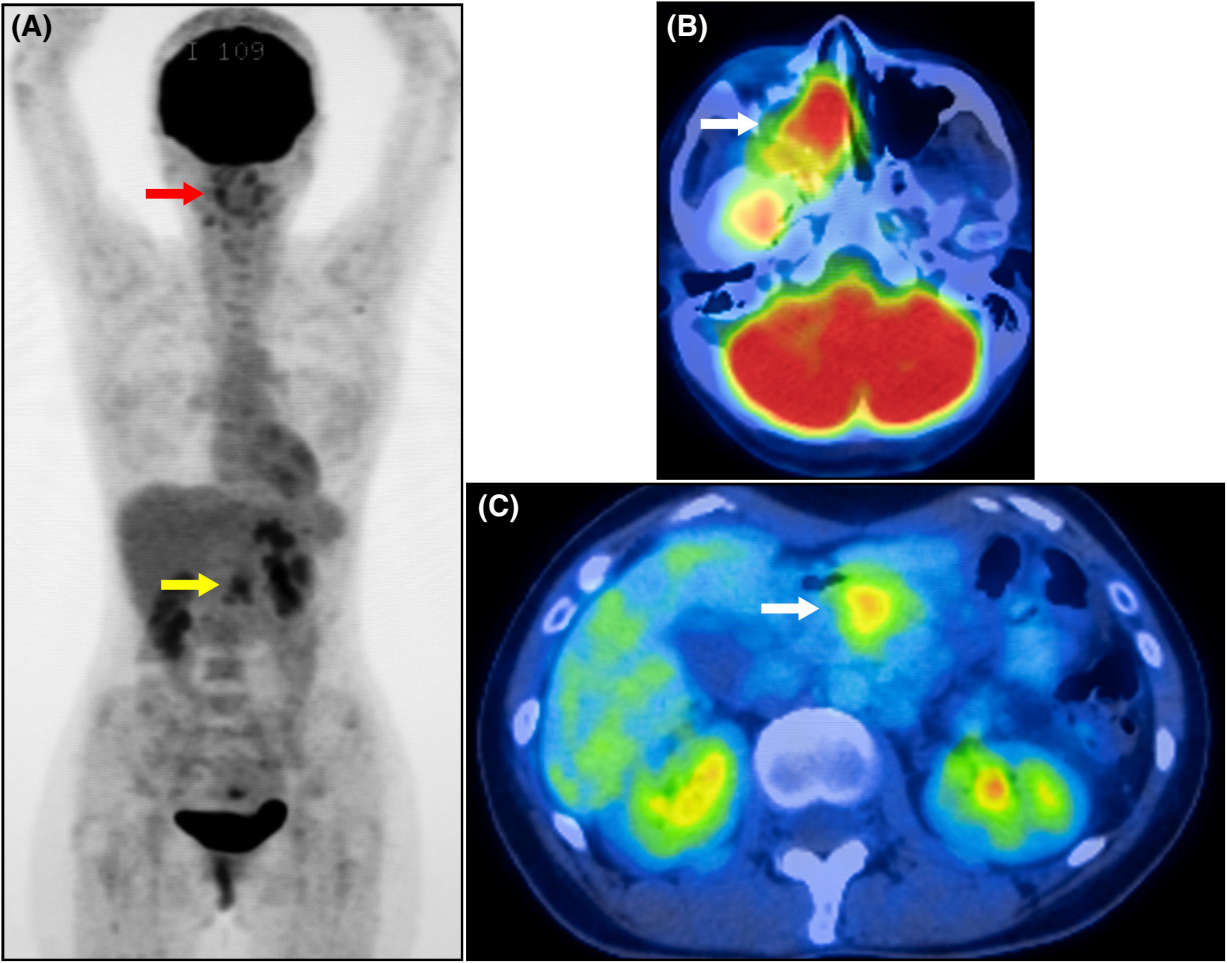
Positron emission tomography‐computed tomography. (A) The maximum intensity projection image of the whole body revealed increased tracer uptake in the right perinasal region (red arrow) and midabdomen (yellow arrow). Transaxial‐fused images revealed hypermetabolic masses (white arrows) in the right ethmoid sinus (B) and pancreatic body (C)
